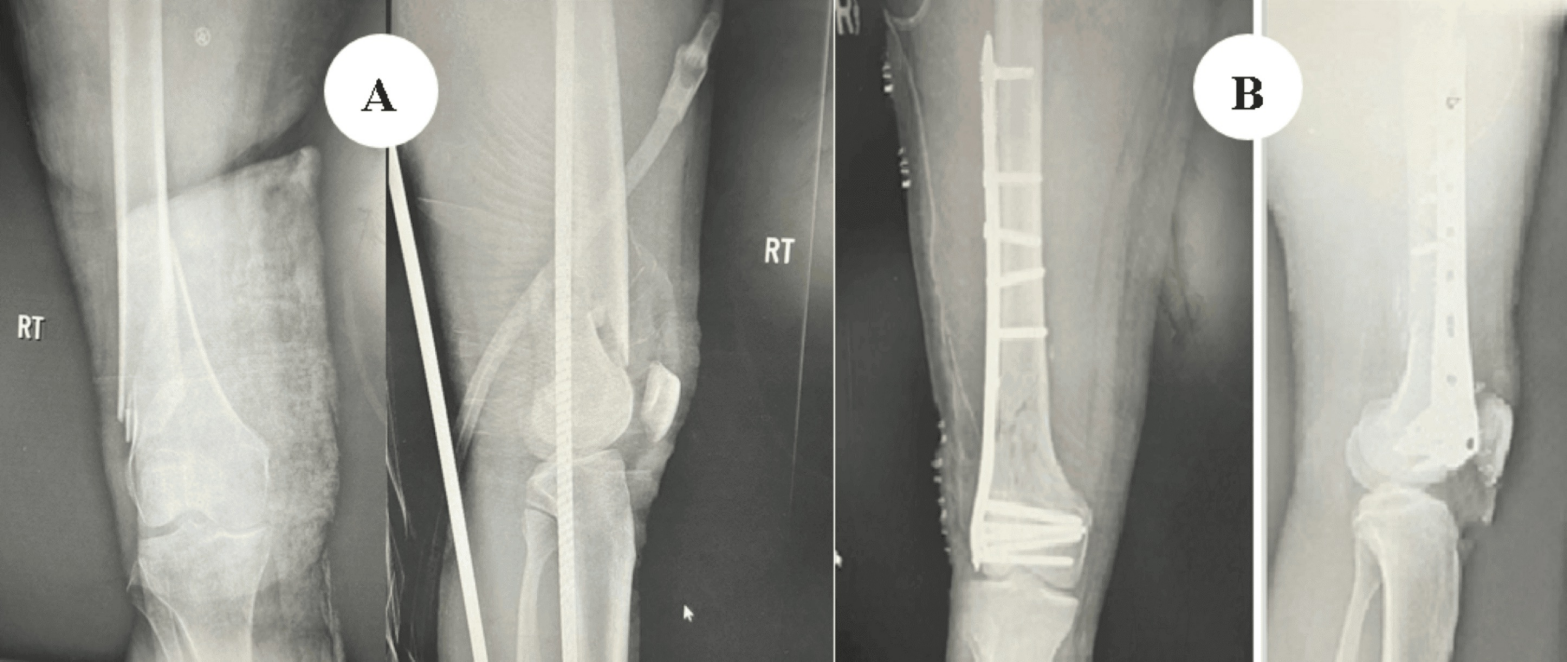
Case 1 (A) preoperative anteroposterior and lateral X-ray views and (B) immediate postoperative X-ray views
